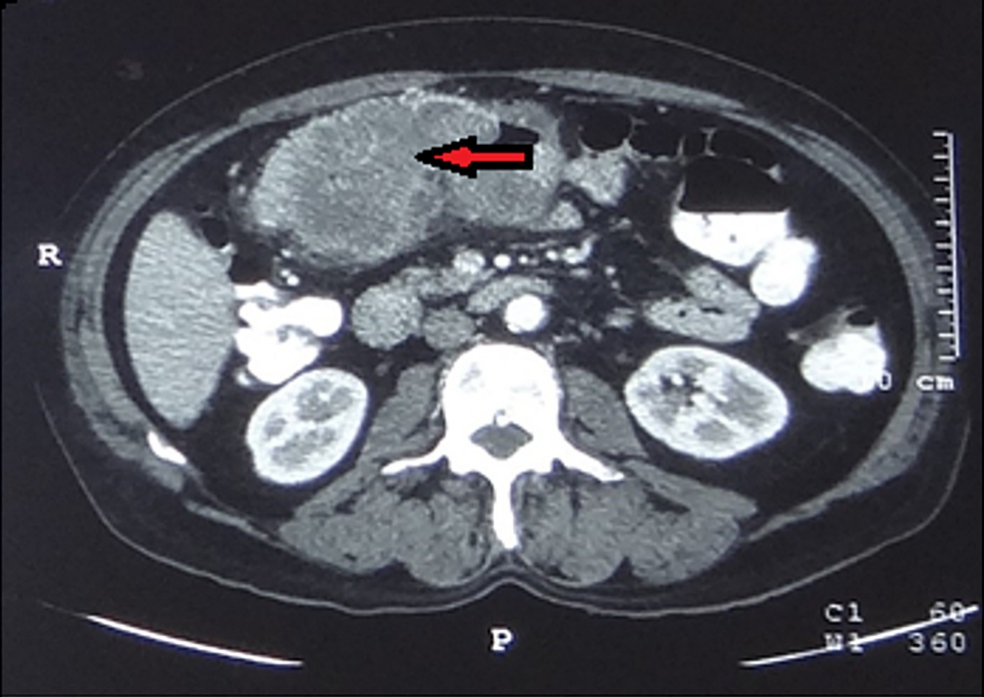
Preoperative CECT abdomen showing enhancing heterogenous transverse colon mass (arrow) CECT: contrast-enhanced computed tomography
